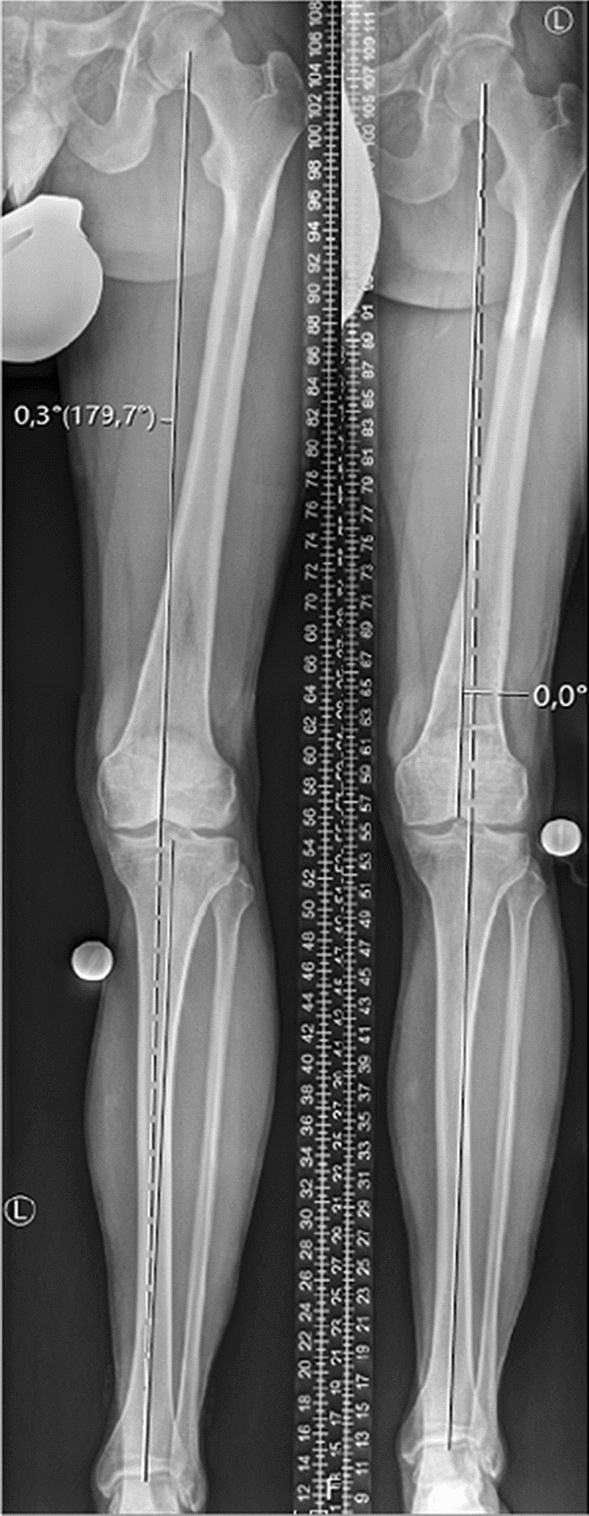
Weight-bearing whole-leg anteroposterior radiographs taken pre- (left) and postoperatively (right) following a derotational distal femoral osteotomy showing no relevant change in coronal limb alignment
